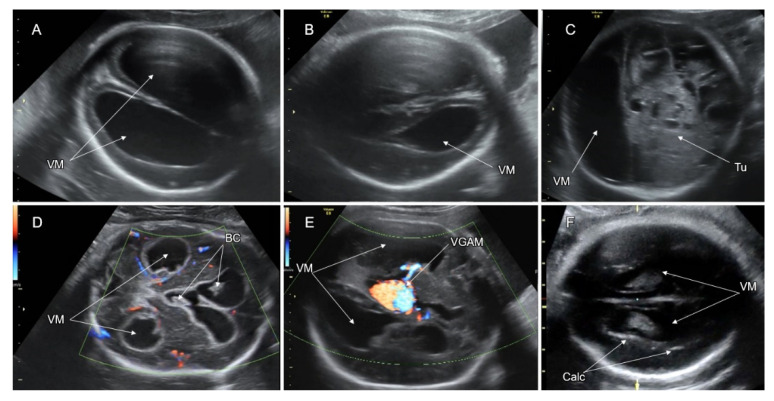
Fetal ventriculomegaly and associated disorders. Legend: (A–F) Fetal head planes on axial sonography: (A) A fetus at 31 weeks of gestation with global severe ventriculomegaly (VM) due to aqueductal stenosis. Note the thin and regular ependymal lining; the third ventricle is dilated (not shown). (B) A fetus at 29 gestational weeks with complete agenesis of the corpus callosum. Note the colpocephalic dilatation of the atrium and occipital horn (VM). (C) A fetus at 26 gestational weeks with a huge intracranial teratoma (Tu). Note a prominent mass effect resulting in midline displacement and severe contralateral ventriculomegaly (VM). (D) A fetus at 36 gestational weeks with intraventricular hemorrhage grade-3. Note a thick and irregular ependymal lining, dilated third ventricle, and intraventricular blood clots (BC). (E) A fetus at 34 gestational weeks with a vein of Galen aneurysmal malformation (VGAM). Note severe bilateral ventriculomegaly (VM) due to the mass effect of the VGAM, leading to obstruction of the CSF flow from the third ventricle. (F) A fetus at 36 gestational weeks with intrauterine CMV infection. Note bilateral ventriculomegaly (VM) and diffuse periventricular calcifications (Calc).
